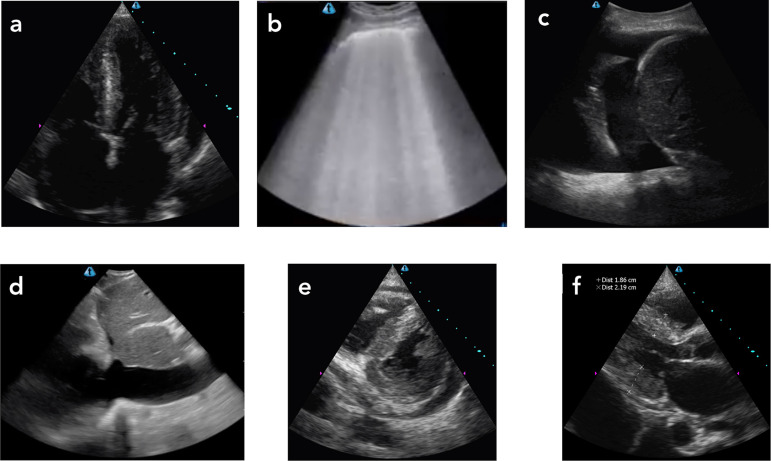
Ultrasound images obtained during the nephrological consultation: a.
